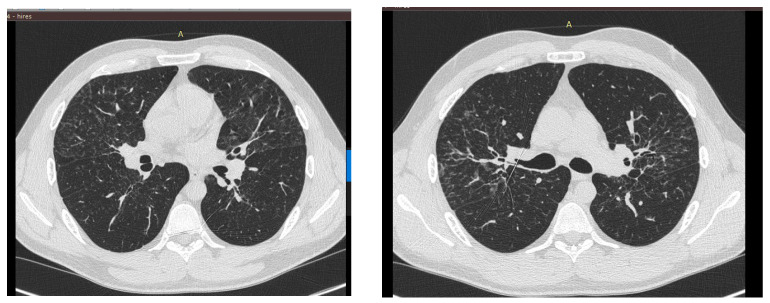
CT of the chest with positive dynamics.
